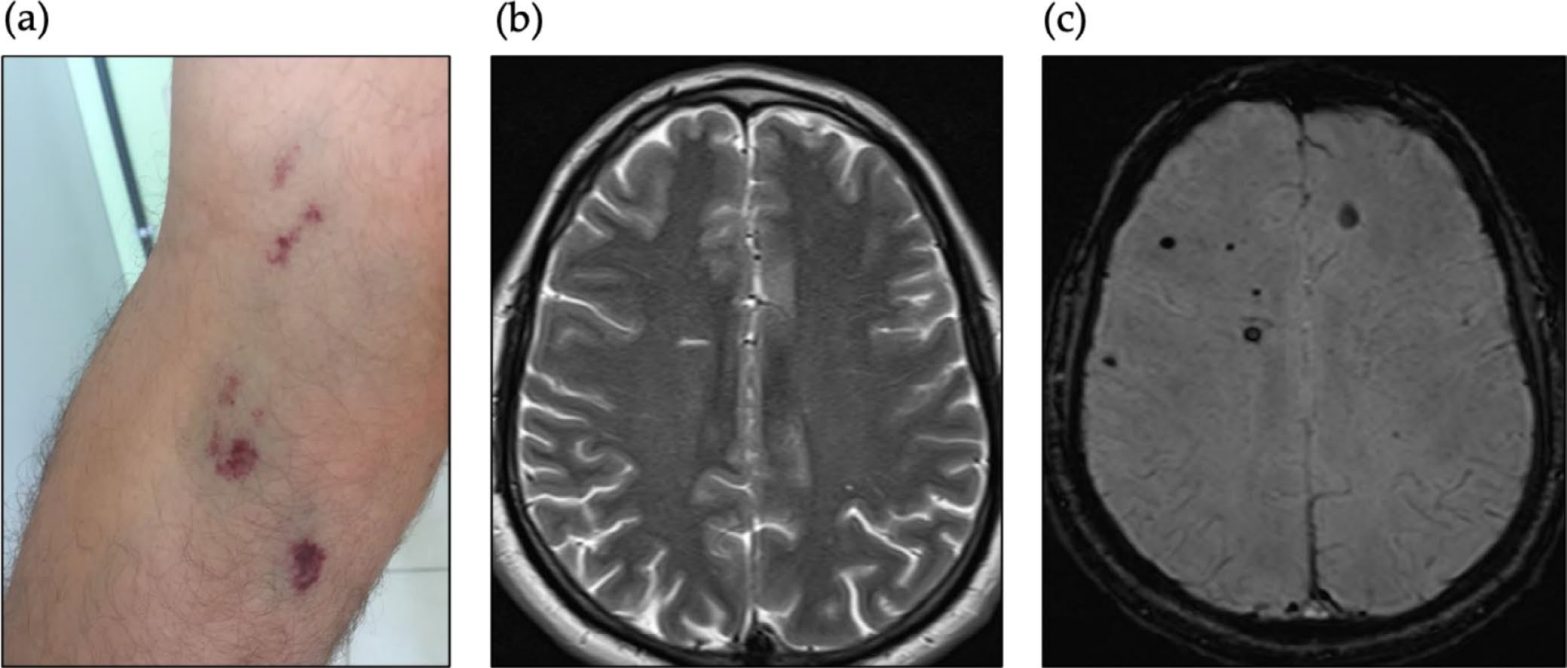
Investigation of familial cerebral cavernous malformation (FCCM) and its clinical features: (a) A 42‐year‐old asymptomatic man, whose mother is known to have multiple brain lesions, presented with multiple violaceous cutaneous lesions on the right forearm. (b) T2‐weighted axial sequence magnetic resonance imaging (MRI) of a very small lesion on the deep left parietal region of a 42‐year‐old asymptomatic man with a mother known to have multiple brain lesions. (c) A susceptibility‐weighted imaging (SWI) sequence of MRI in the same axial cut as (b), showing multiple lesions.
